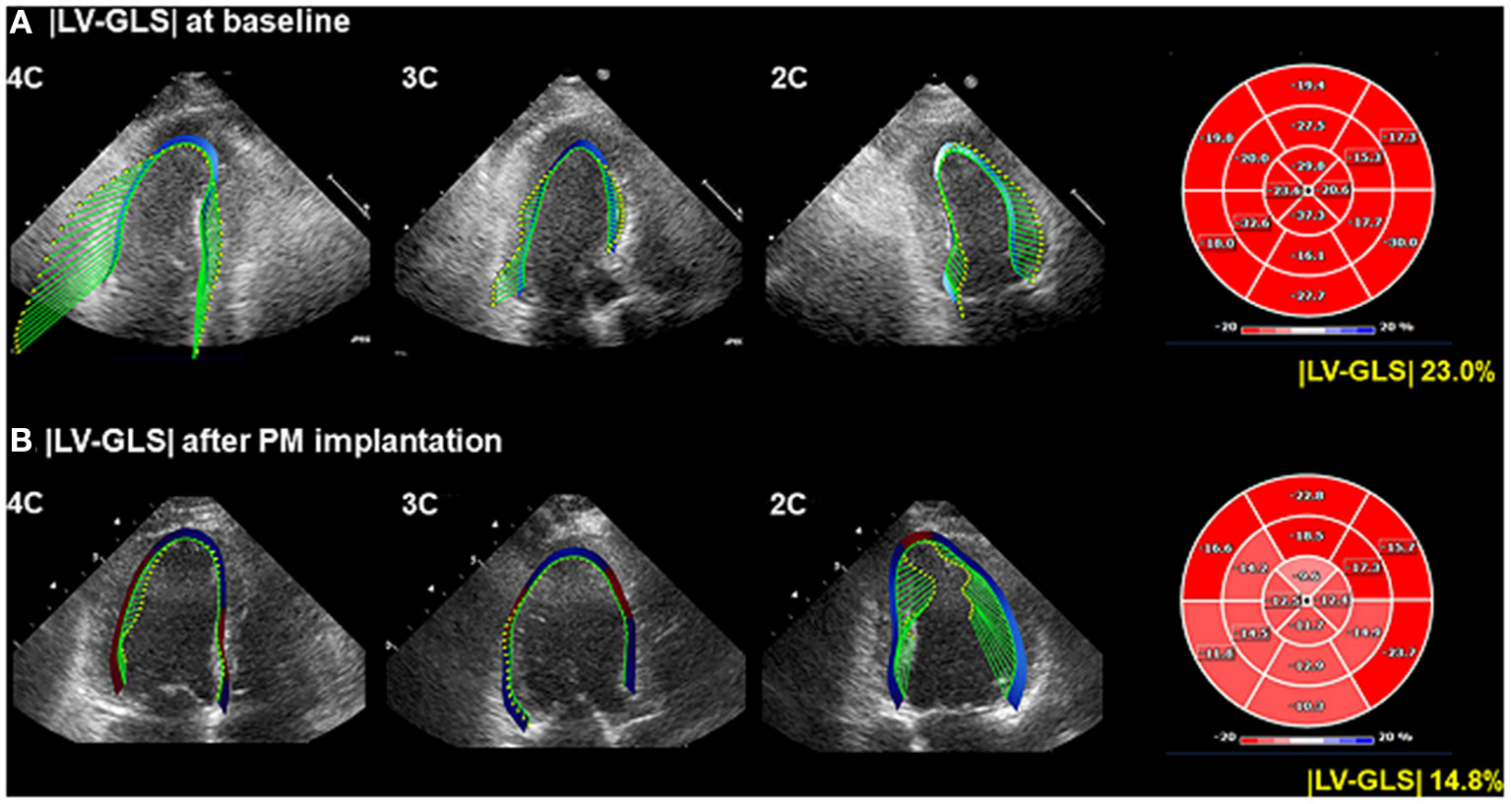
Representative LV-GLS measurements. (A) LV-GLS was calculated from three standard apical views at baseline echocardiogram (left). The absolute value of LV-GLS was 23.0% (right). (B) LV-GLS was assessed after PM implantation. |LV-GLS| was 14.8% (right). LV-GLS, left ventricular global longitudinal strain; PM, pacemaker.
